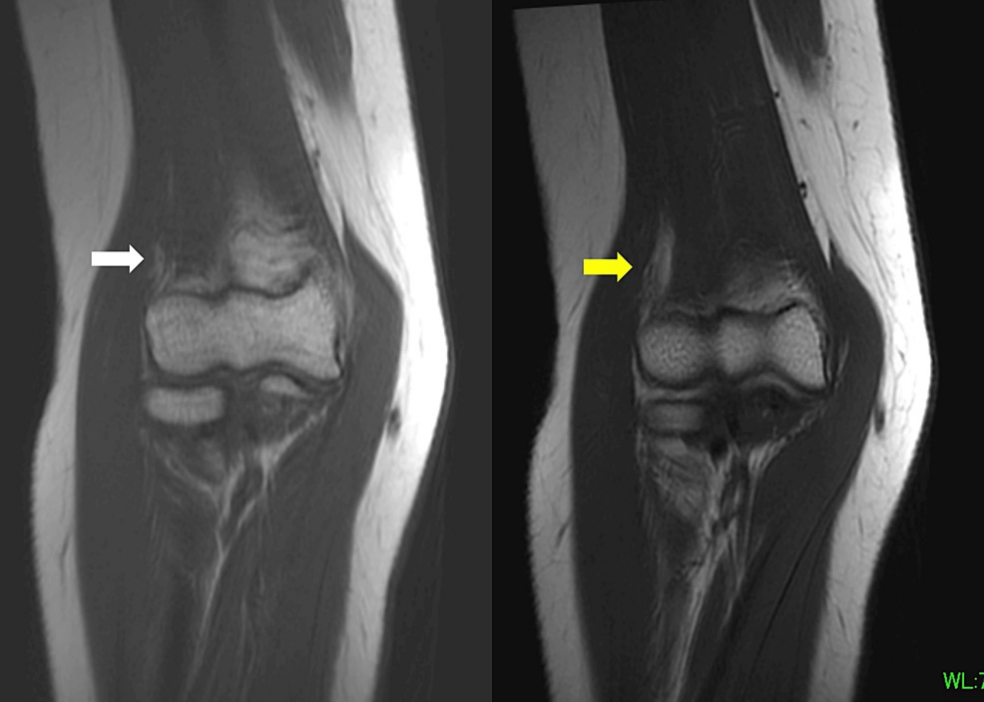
Sagittal view of preoperative MRI. Left side: T1WI. Iso- and high-intensity occupying lesions (white arrow) are seen. Right side: T2WI. High-intensity occupying lesions (yellow arrow) are seen. T2WI, T2-weighted image; T1WI, T1-weighted image
